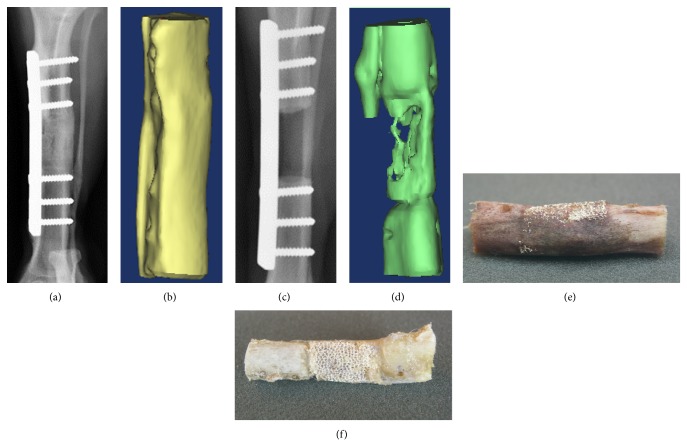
Various images of beagle tibiae with scaffold in defaced sites (20 mm) at 8 weeks following implantation. (a), (b), and (e) Segmental defect site with 3 mm passage in a scaffold. (c), (d), and (f) Segmental defect site without passage in a scaffold. (a) and (c) Radiographs of segmental defect sites. (b) and (d) 3D CT reconstructed images of segmental defect sites after removal of plate and screws at autopsy. (e) and (f) Gross images of segmental defect sites.
